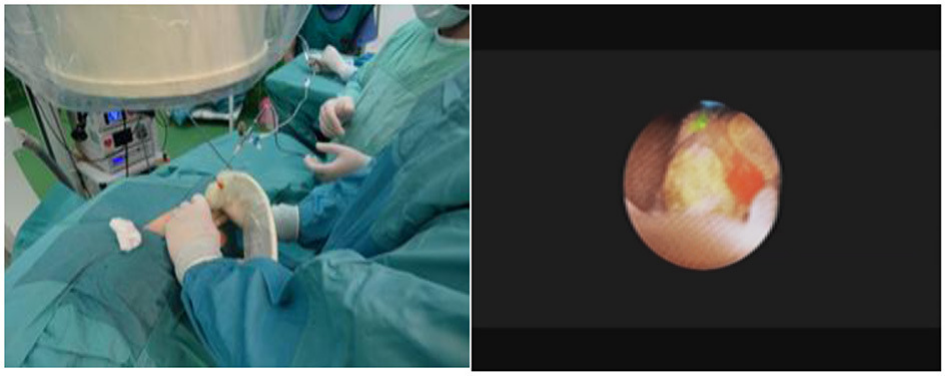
Kidney puncture under ultrasound control. Stone in the “all-seeing needle” view.
